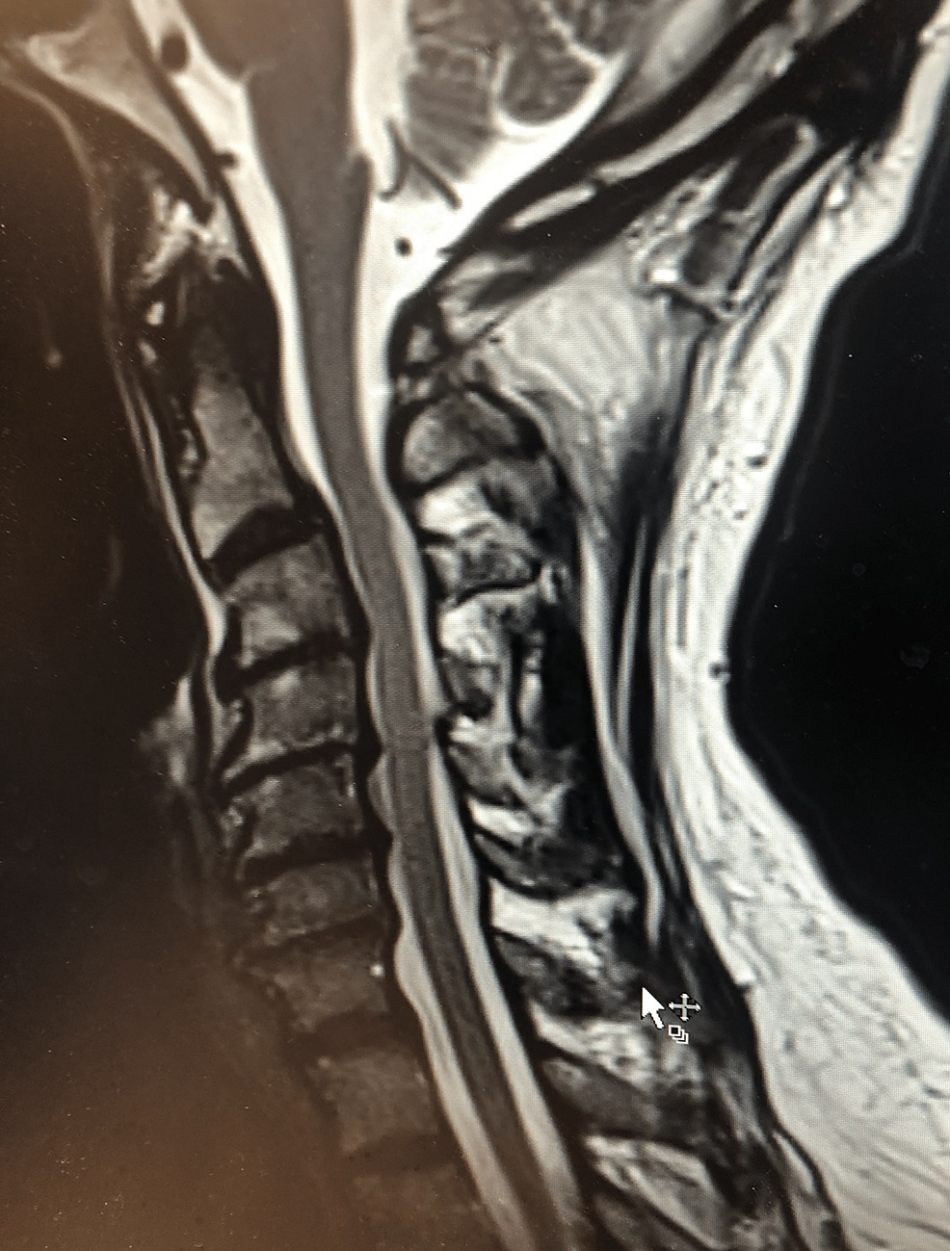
Preoperative sagittal T2-weighted MRI. This is a preoperative MRI of the cervical spine demonstrating spondylosis between C3 and C7. MRI, magnetic resonance imaging
